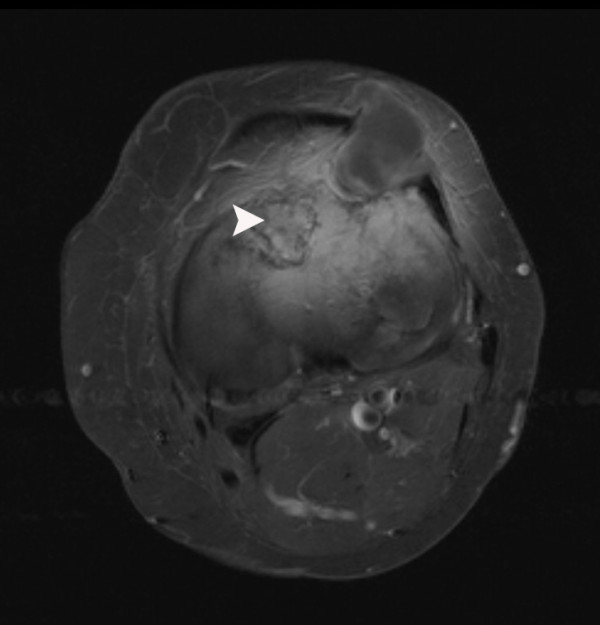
T2-weighted MRI scan of our patient. This T2-weighted image shows identical thin rim enhancement and heterogeneous intra-lesional enhancement in both masses. These findings may suggest myxomatous masses. In contrast, fibrous dysplasia can be noticed in the anterior tibial plateau (arrowhead).
